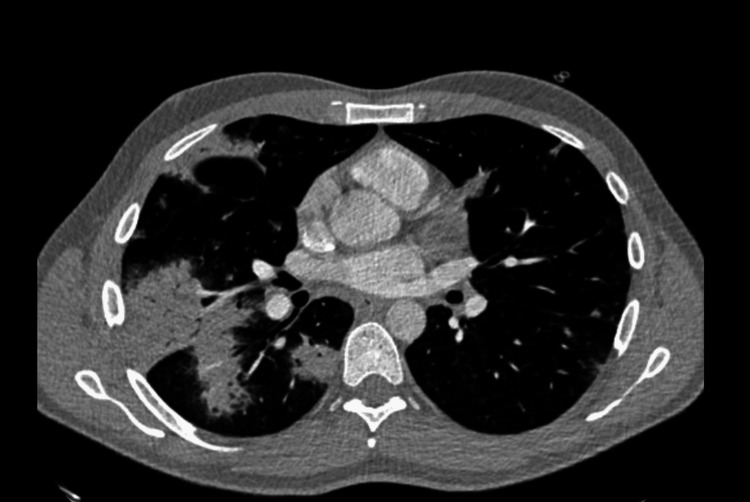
Chest CT scan performed in ED.
